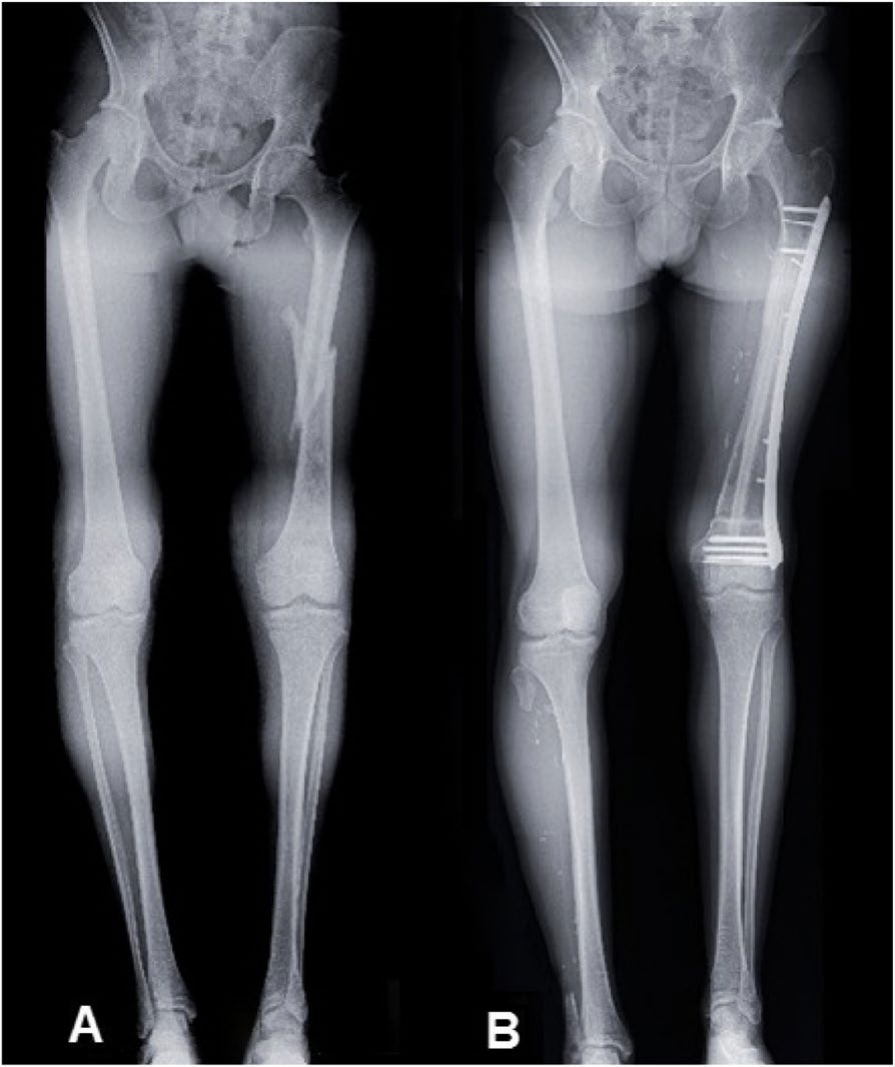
Intercalary femoral reconstruction for a high-grade fracture osteosarcoma using an allograft associated with a vascularised fibula graft and an LCP bridging plate. A Pre-operative pangonogram X-ray view. B Two-year follow-up X-ray view
